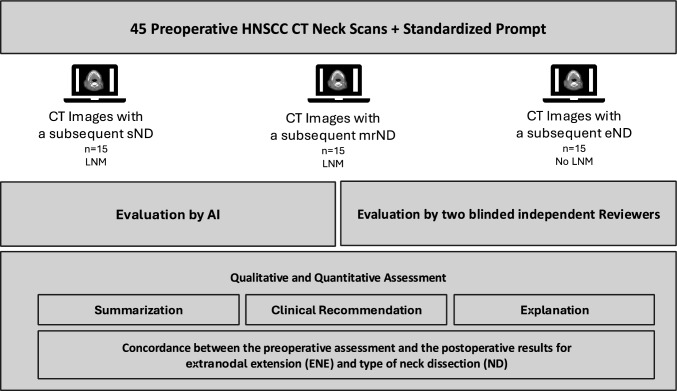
Depiction of the preoperative CT scans before a mrND, sND or eND and the corresponding interpretation by ChatGPT-4V. The sagittal, coronal and axial images are depicted. The answers were copied from the response page
